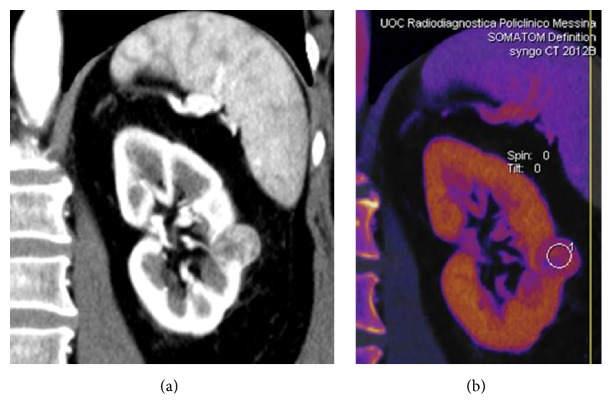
Small cc-RCC. Coronal reformatted DE arterial-phase CT image (a); coronal color-coded iodine overlay image (b). High enhanced solid renal mass with an high iodine content (2.4 mgI on ROI).
